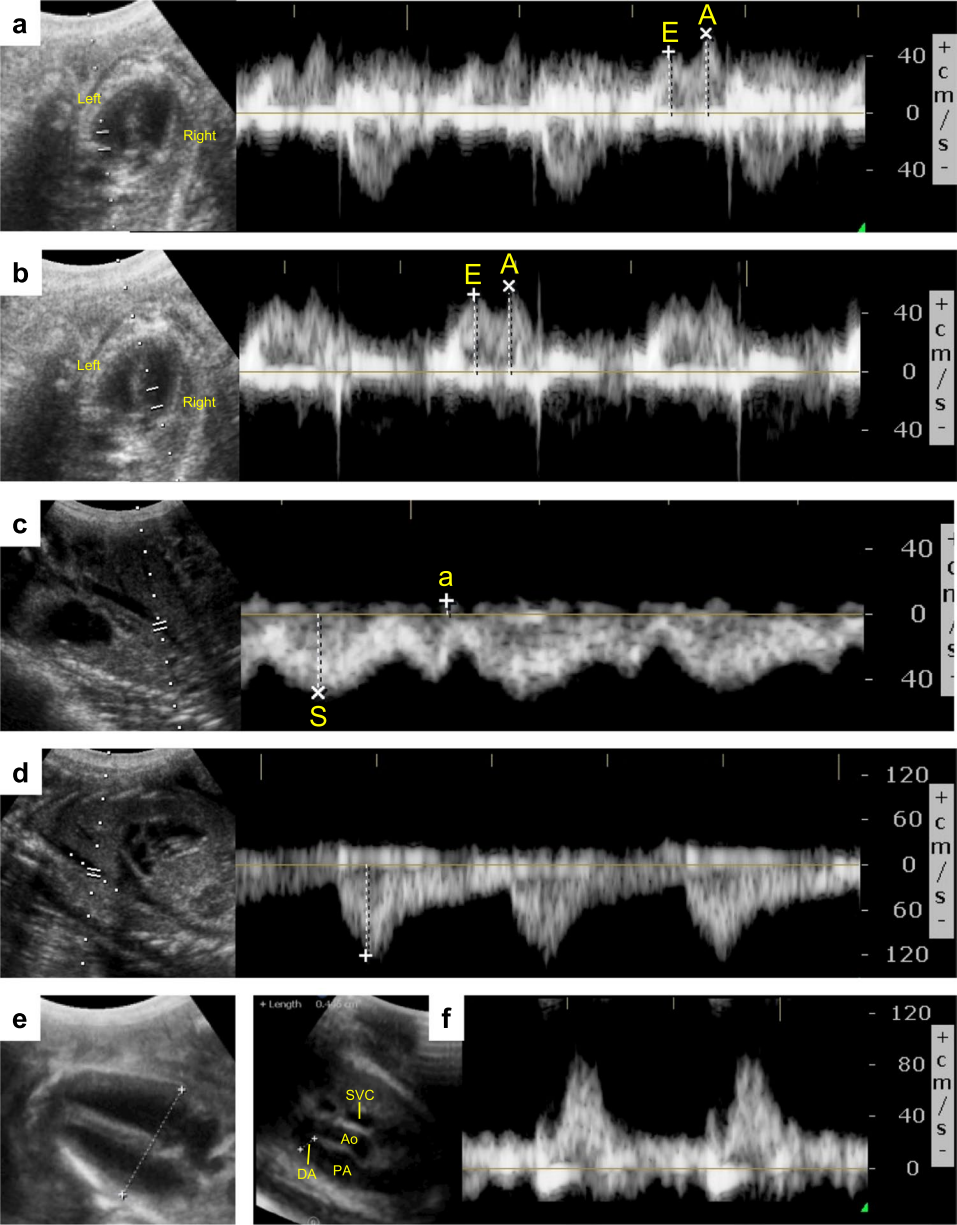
Representative images of fetal cardiac ultrasound. Cross-sectional view showing 4-chamber view (a, b, and e), sagittal view (c and d), and three-vessel trachea view (f). (A and B) Doppler waveforms across the mitral (a) and tricuspid (b) valves during diastole. In the biphasic doppler waveforms, E corresponds to early diastolic peak velocity and A corresponds to atrial contraction peak velocity. The E/A ratio is used for Doppler waveform quantification across the atrioventricular valves. c Doppler waveform of inferior vena cava. S represents maximum systolic velocity, and a represents the atrial reversal flow during atrial contraction. The a/S ratio was calculated to obtain the preload index. d Doppler waveform of descending aorta (dAo) at the level of the diaphragm. The maximum systolic blood velocity (Vmax) was measured. e Distance between the attachment point of the mitral valve on the epicardium to the attachment point of tricuspid valve on the epicardium was measured in the 4-chamber view as total cardiac dimension. f Doppler waveform of the ductus arteriosus. SVC, superior vena cava; Ao, aorta; PA, pulmonary artery
